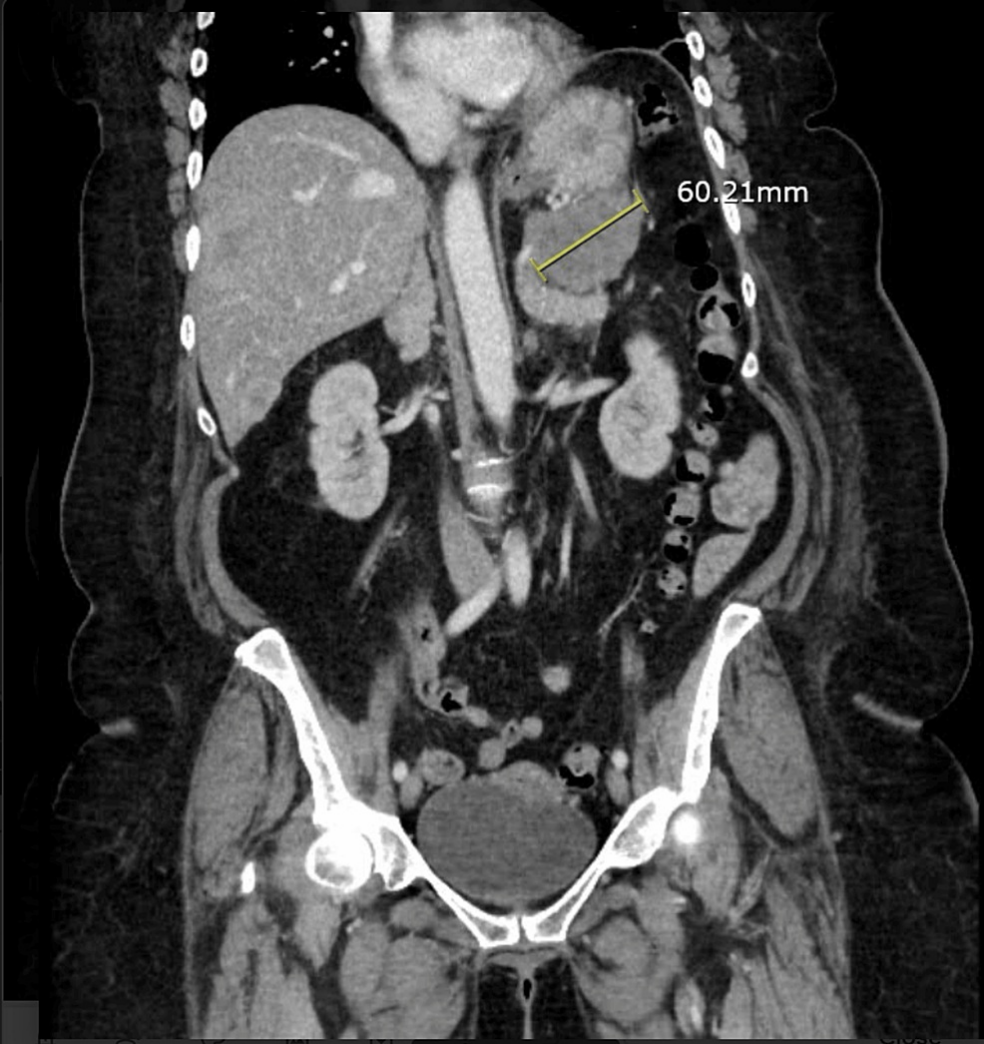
CT chest, abdomen, and pelvis frontal section displaying a 6.0 cm pancreatic tail mass with multiple liver metastases
